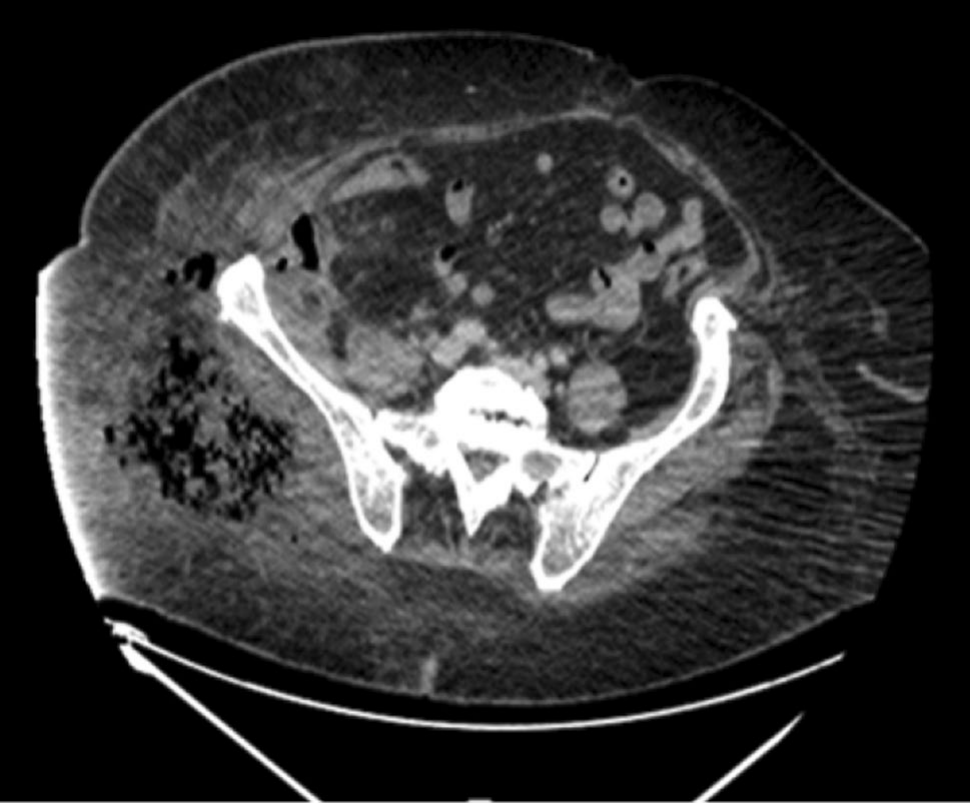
A 43-years-old male operator who sustained a high-pressure water jet injury in the right thigh, where the picture shows a CT-scan at the level of thigh and pelvis. There is a large right gluteal collection of gas bubbles associated with inflammation and edema extending to the lateral abdominal wall subcutaneous tissues. A smaller complex air-fluid collection is also noted deep to the lateral lower abdominal wall and in the right iliacus muscle
